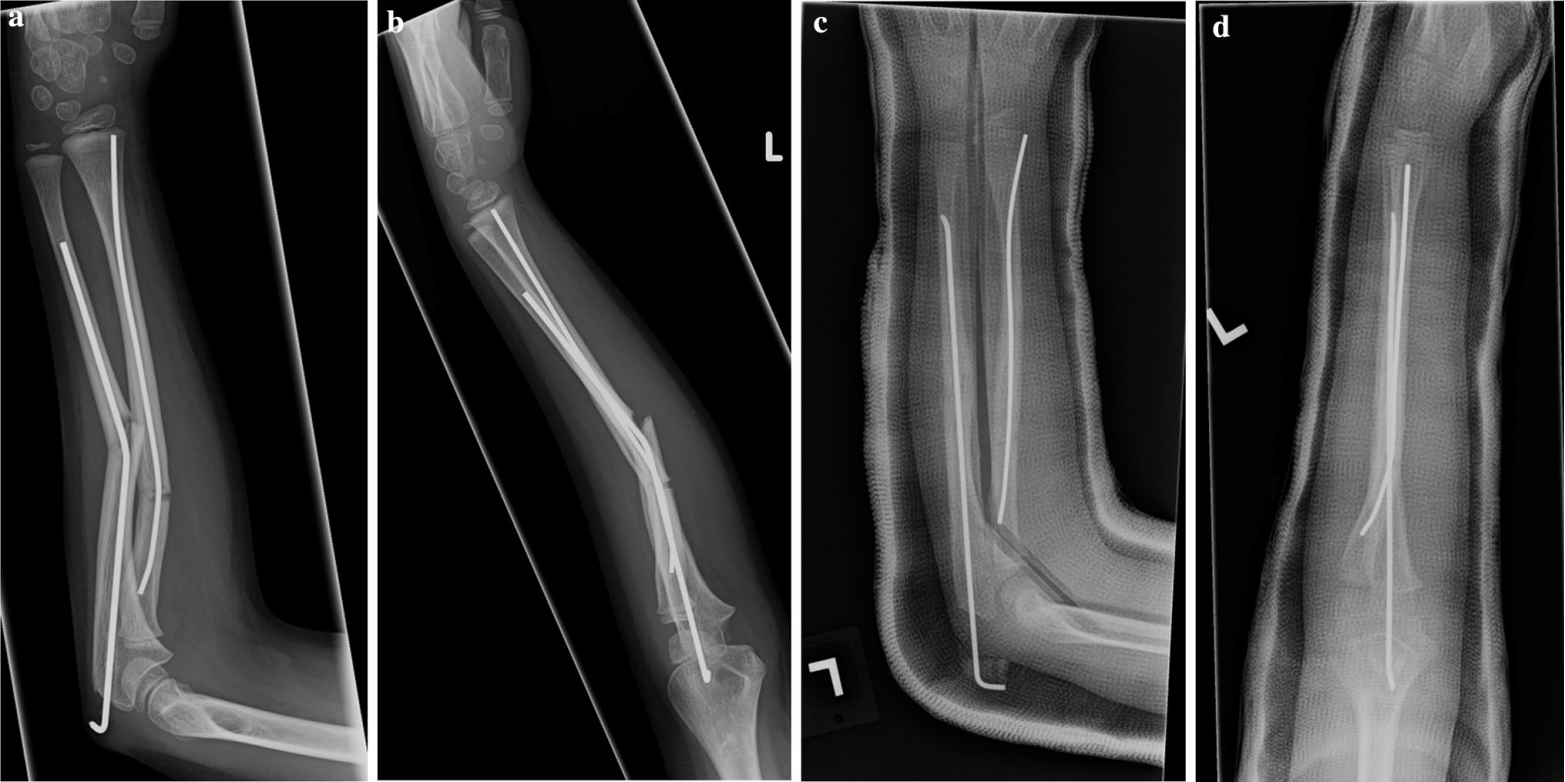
Anteroposterior (a) and lateral (b) radiographs of a 6 year old girl (Patient 1) who sustained a refracture after a trip and fall. She was treated with rebending of the radial implant and removal and replacement of the ulnar implant (c, d)
